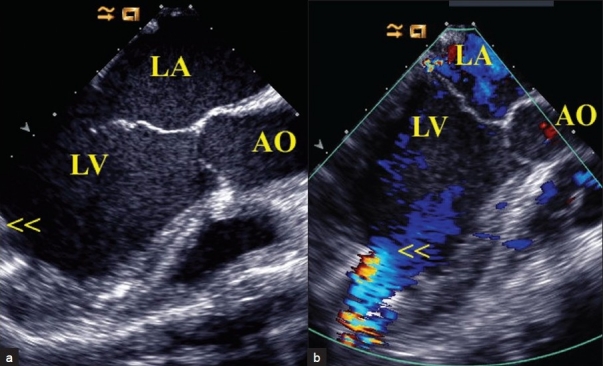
(a) Intraoperative tranesophageal echocardiography at 115° showing the LVAD inflow conduit from the LV apex (arrows) and (b) the color flow in the conduit (arrows)
